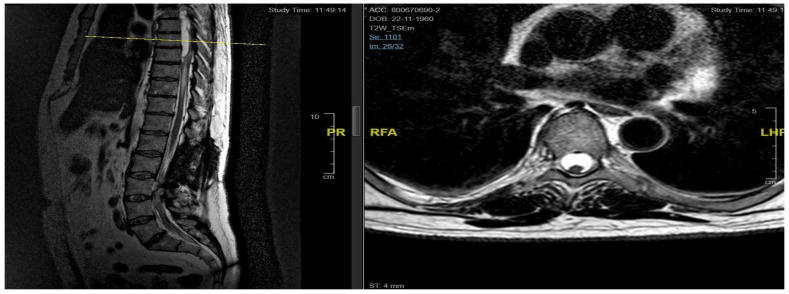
Case 3: pre-operative MRI showing an intradural and extramedullary cyst: pre-operative T2 sagittal view (left) and pre-operative T2 axial view (right).
